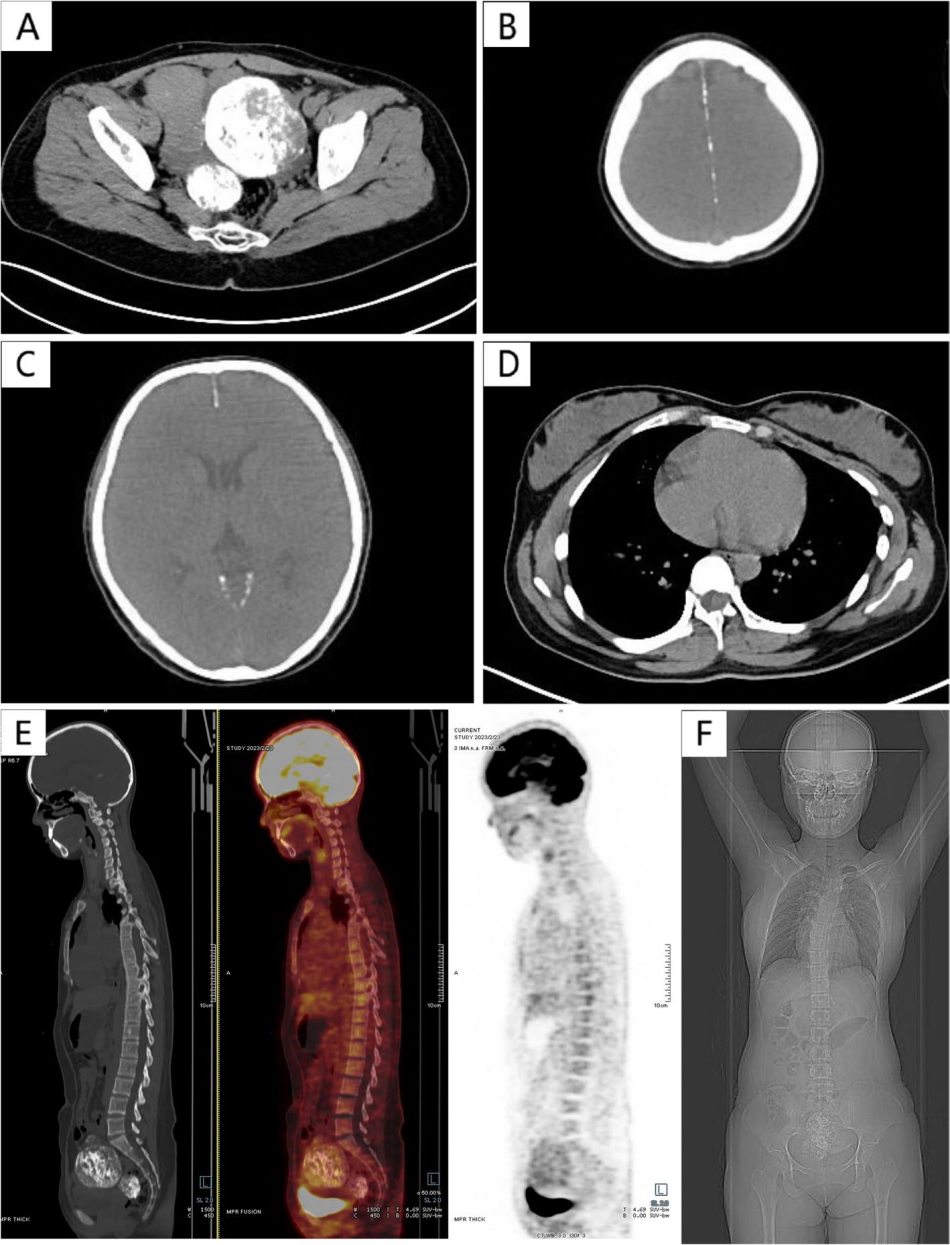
PET/CT imaging. A Axial CT showing multiple high-density nodular masses in the lower abdomen and pelvic cavity with multiple calcifications. B Axial CT showing extensive nodular calcifications in the falx cerebri. C Axial CT showing extensive nodular calcifications in the tentorium cerebelli. D Axial CT showing asymmetry in the thorax with a slight collapse in the left side. E Mildly increased uptake of FDG within the pelvic masses. F X-ray posteroanterior, chest view, showing scoliosis of thoracic spine
